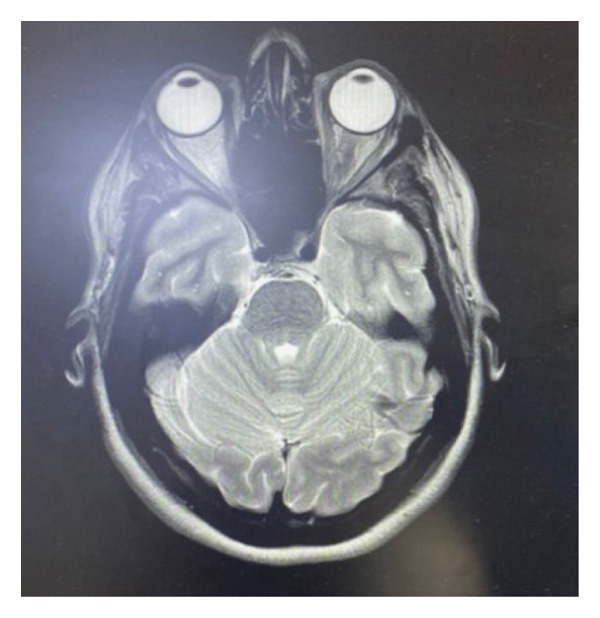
An MRI shows a complete resolution of the previous orbital tumor on brain MRI.
